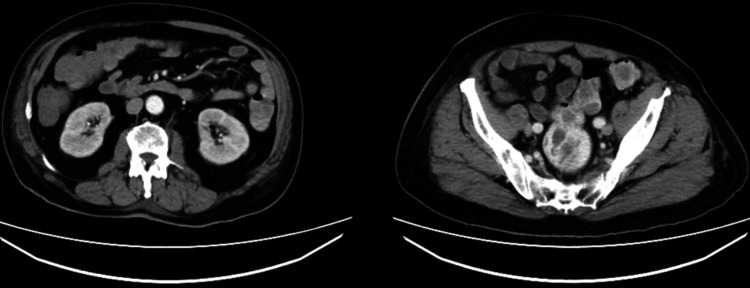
Abdominal CT reveals no active bleeding
